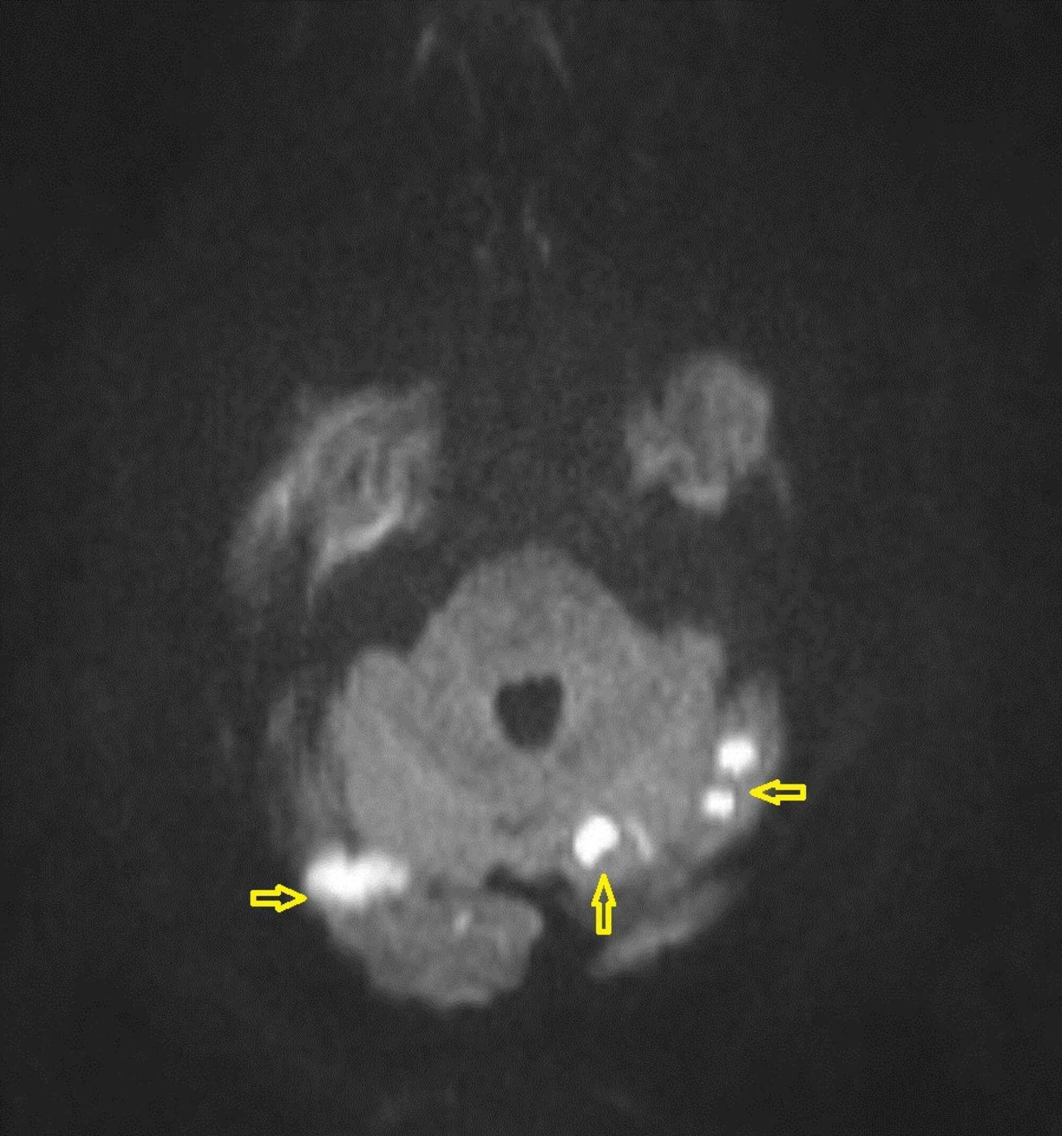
MRI Brain showed multiple small acute infarcts involving bilateral posterior cerebral arteries distribution
